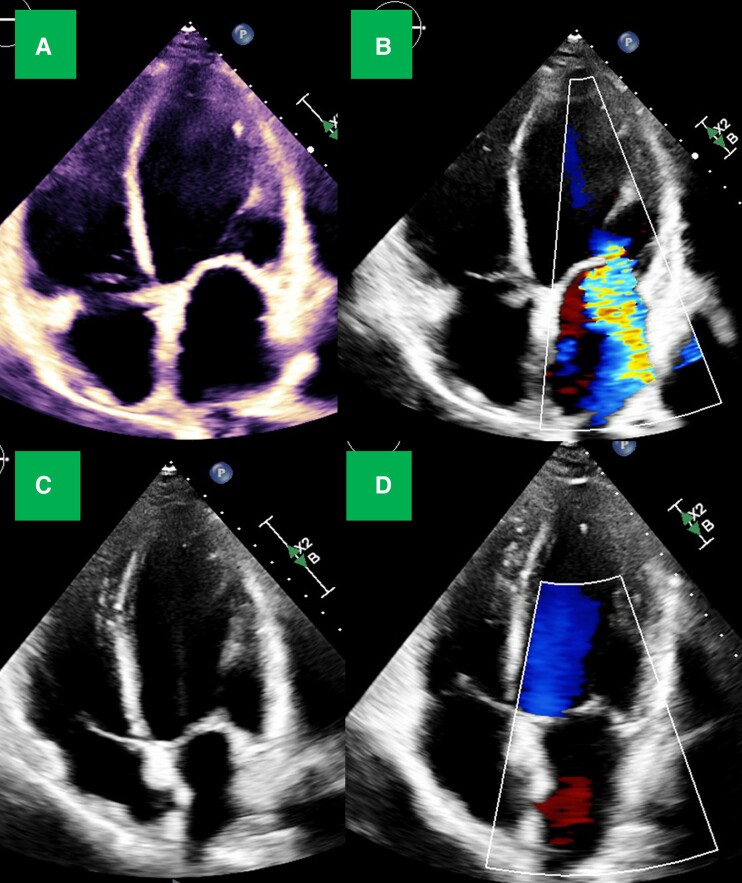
Patient 2 echocardiogram. Admission: (*A*) end-diastole, 270 mL, (*B*) systole with severe mitral regurgitation 6 months later: (*C*) end-diastole, 155 mL, (*D*) systole with no mitral regurgitation.
